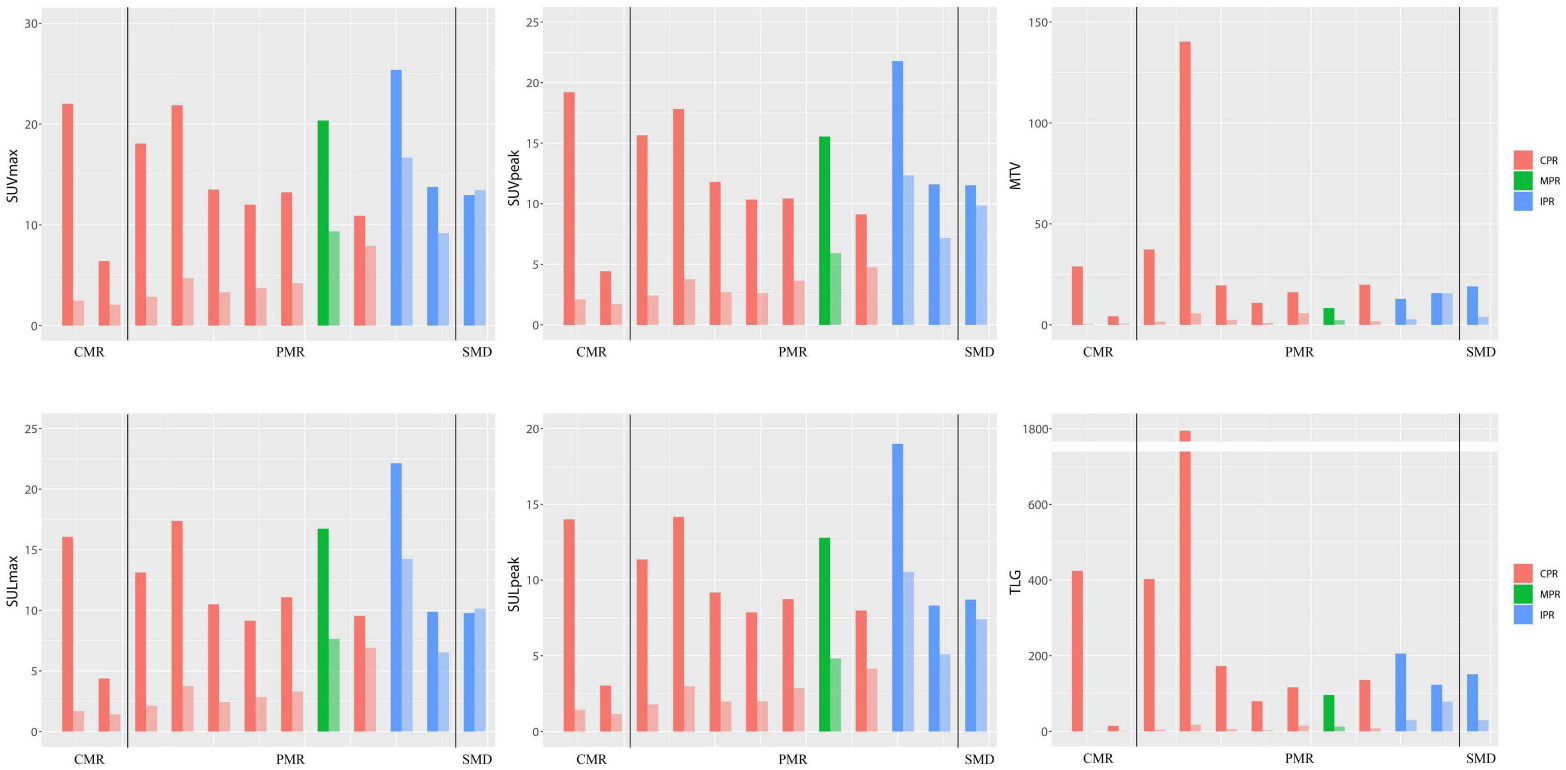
Bar plot for six SUV statistics features of paired PET (baseline and preoperative). The dark colors represent baseline PET features, and the light colors represent preoperative PET features. The patient number from left to right is 16, 5, 21, 23, 4, 12, 20, 19, 1, 14, 18, and 22, respectively. CMR, complete metabolic response; PMR, partial metabolic response; SMD, stable metabolic disease; CPR, complete pathological response; MPR, major pathological response; IPR, incomplete pathological response.
